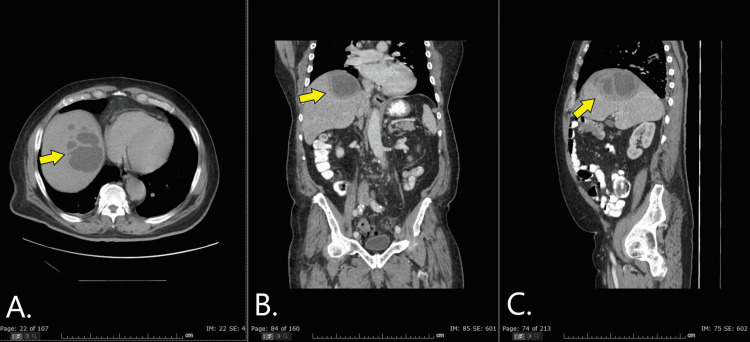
Axial (A), coronal (B), and sagittal (C) views of CT abdomen/pelvis with contrast The images depict the liver lesion one month after initial CT scan - now measuring 10 cm in diameter and with multiple well-defined low-density areas, representing abscess (yellow arrow). Note the characteristic “double target sign” which is best appreciated on the coronal and sagittal views.
